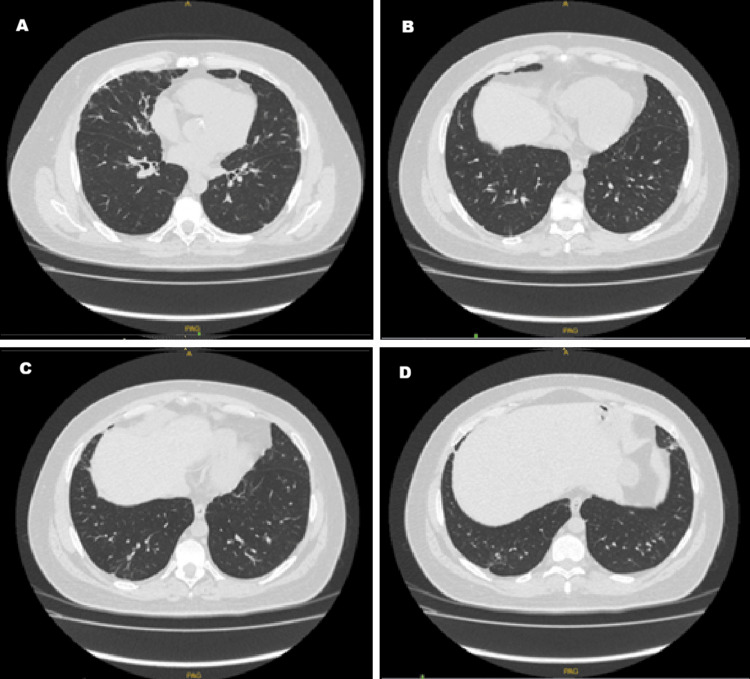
High-resolution computed tomography (HRCT) of the chest, performed five months after initiation of treatment A - D: Resolution of alveolar opacities is observed
